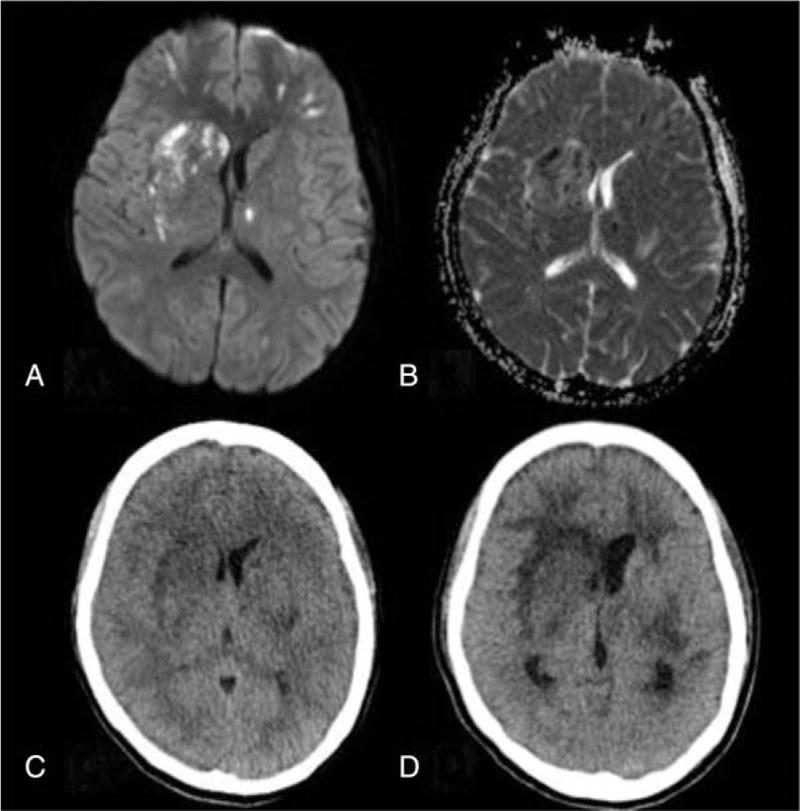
Multiple cerebral infarctions are evident in (A) a diffusion-weighted image, (B) an apparent diffusion coefficient map; brain CT scan showing progressive edema on postoperative days 4 (C) and 7 (D). CT = computed tomography.
